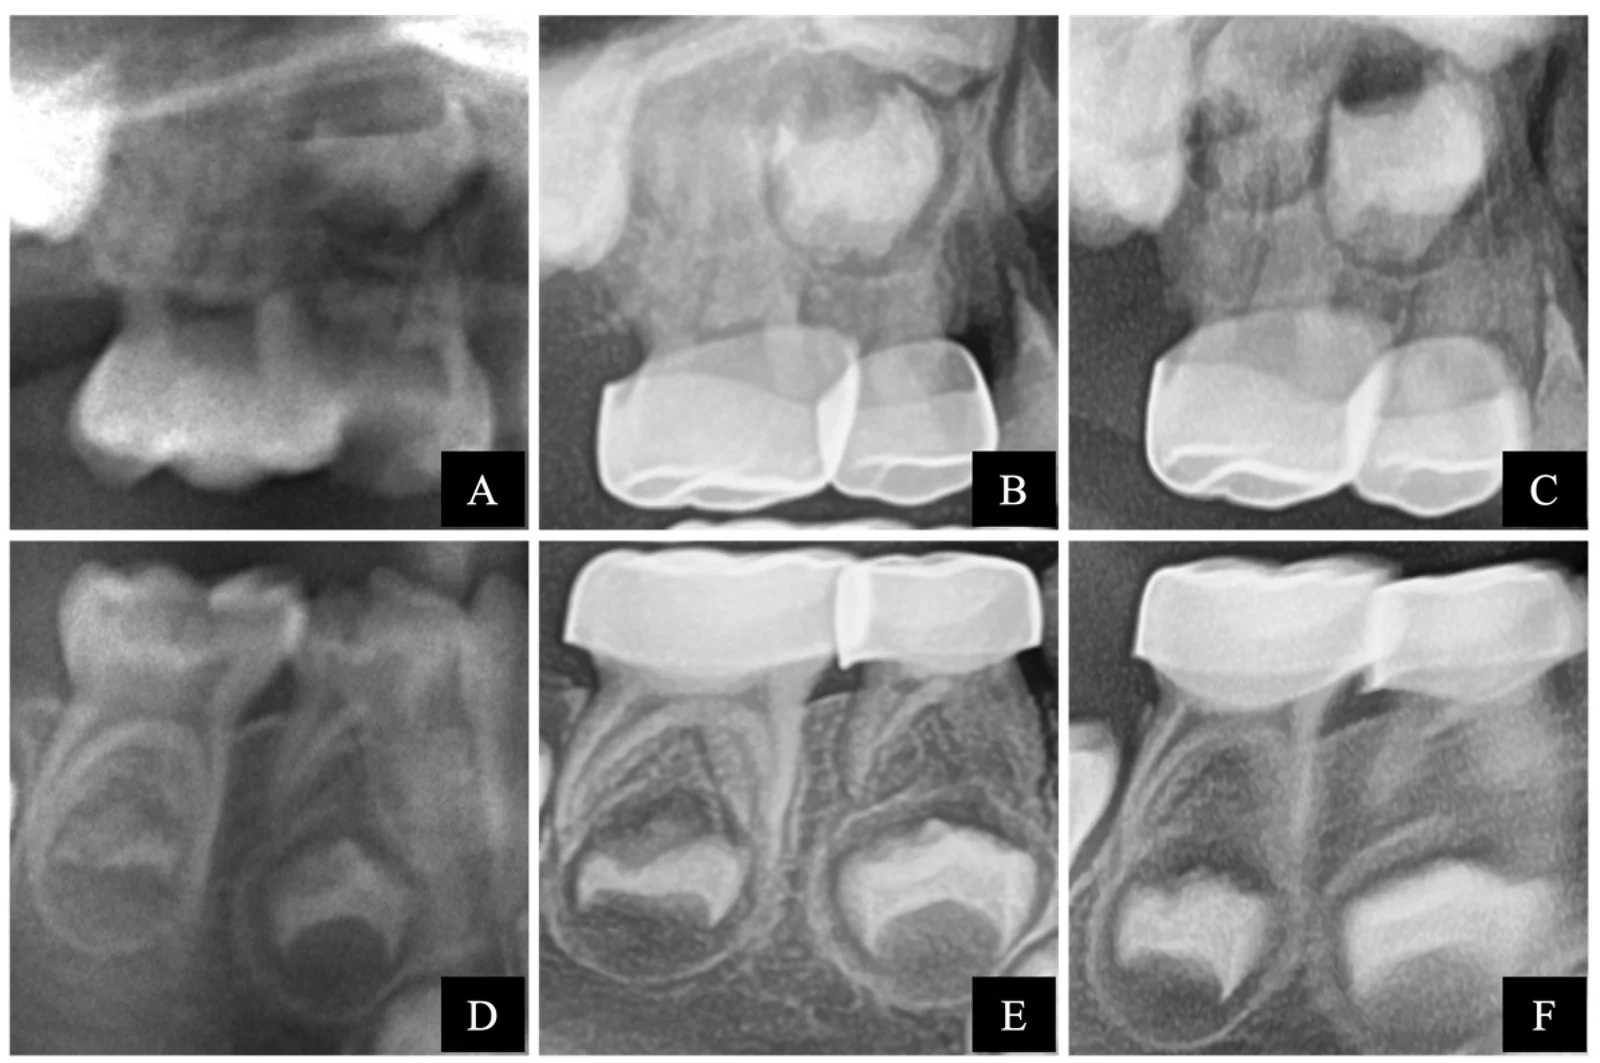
Radiographic evaluation of teeth 54, 84, and 85 in Patient 3. (A–C) Tooth 54: (A) Preoperative radiograph; (B) 6-month post-treatment radiograph; (C) final follow-up radiograph. (D–F) Teeth 84 and 85: (D) Preoperative radiograph; (E) 6-month post-treatment radiograph; (F) final follow-up radiograph.
